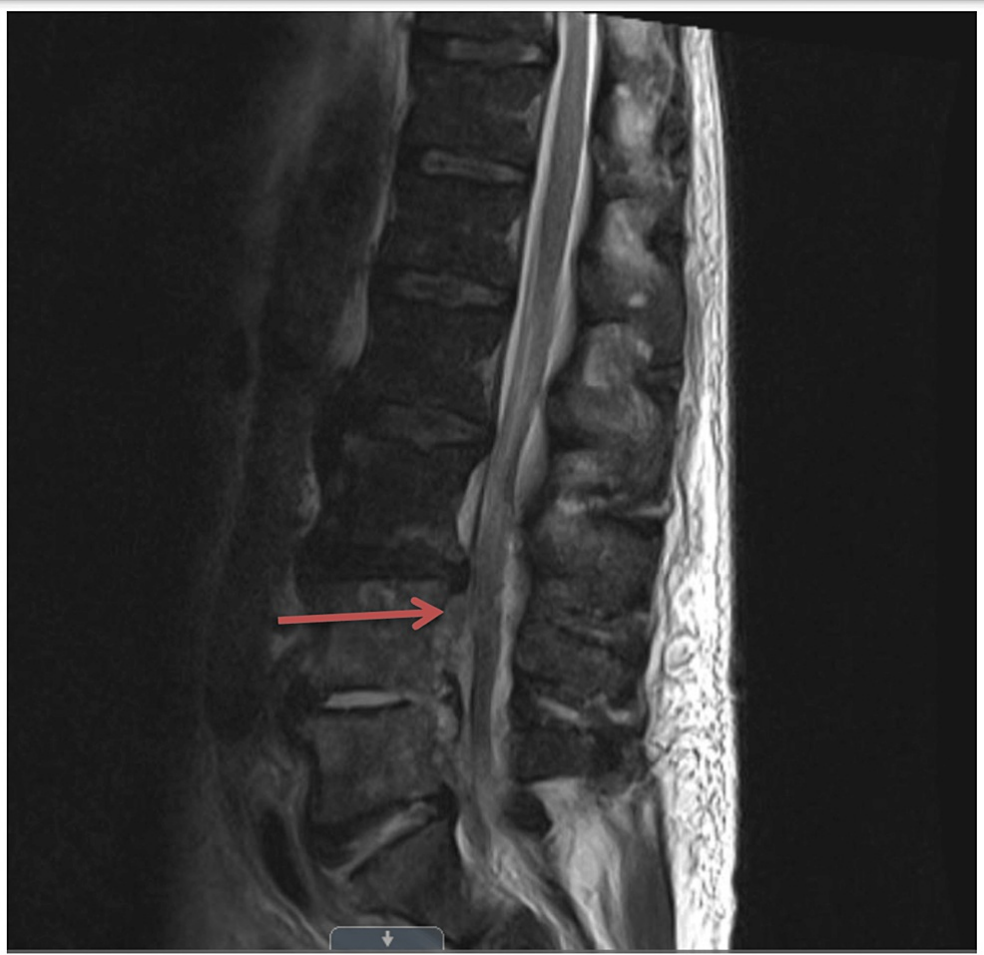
MRI of the lumbar spine with gadolinium contrast shows significant discitis at L4-L5 and L5-S1 with epidural phlegm causing considerable mass effect on the thecal sac (red arrow).
